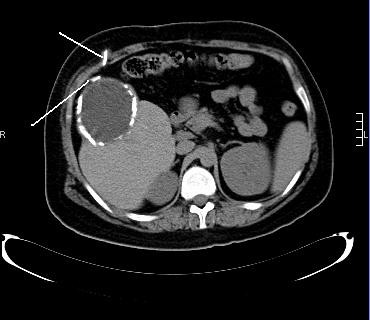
Abdominal CT scan showing 11 × 9 cm pseudocyst of his right hepatic lobe, with peripheral calcifications and the tip of the VP shunt going inside the cyst (arrow).
